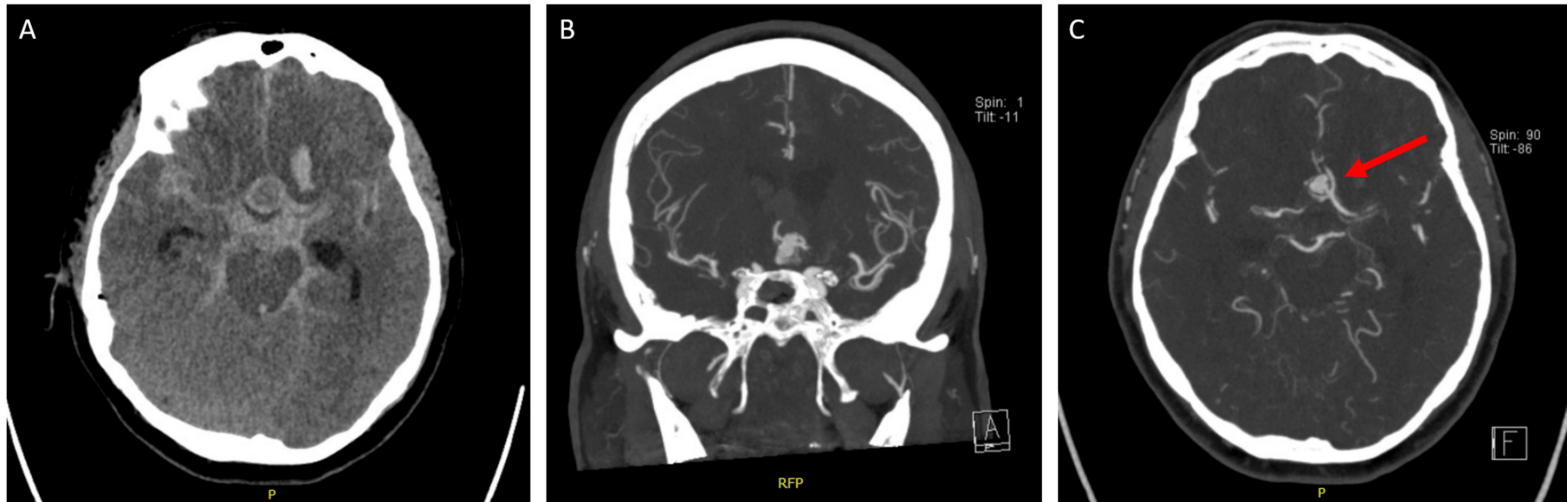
Images of the patient's multiplanar cerebral aneurysm and subarachnoid hemorrhage The images represent different projections from a cerebral computed tomography angiography (CTA) performed on the patient. The axial view (A) reveals an extensive subarachnoid hemorrhage with a potential aneurysmal lesion associated with the anterior communicating artery complex. The coronal view (B) further characterizes an aneurysm or pseudoaneurysm about the same arterial structure. Lastly, the axial image in (C) presents a suggestive view of an aneurysm or pseudoaneurysm with the location of the lesion indicated by the arrow.
